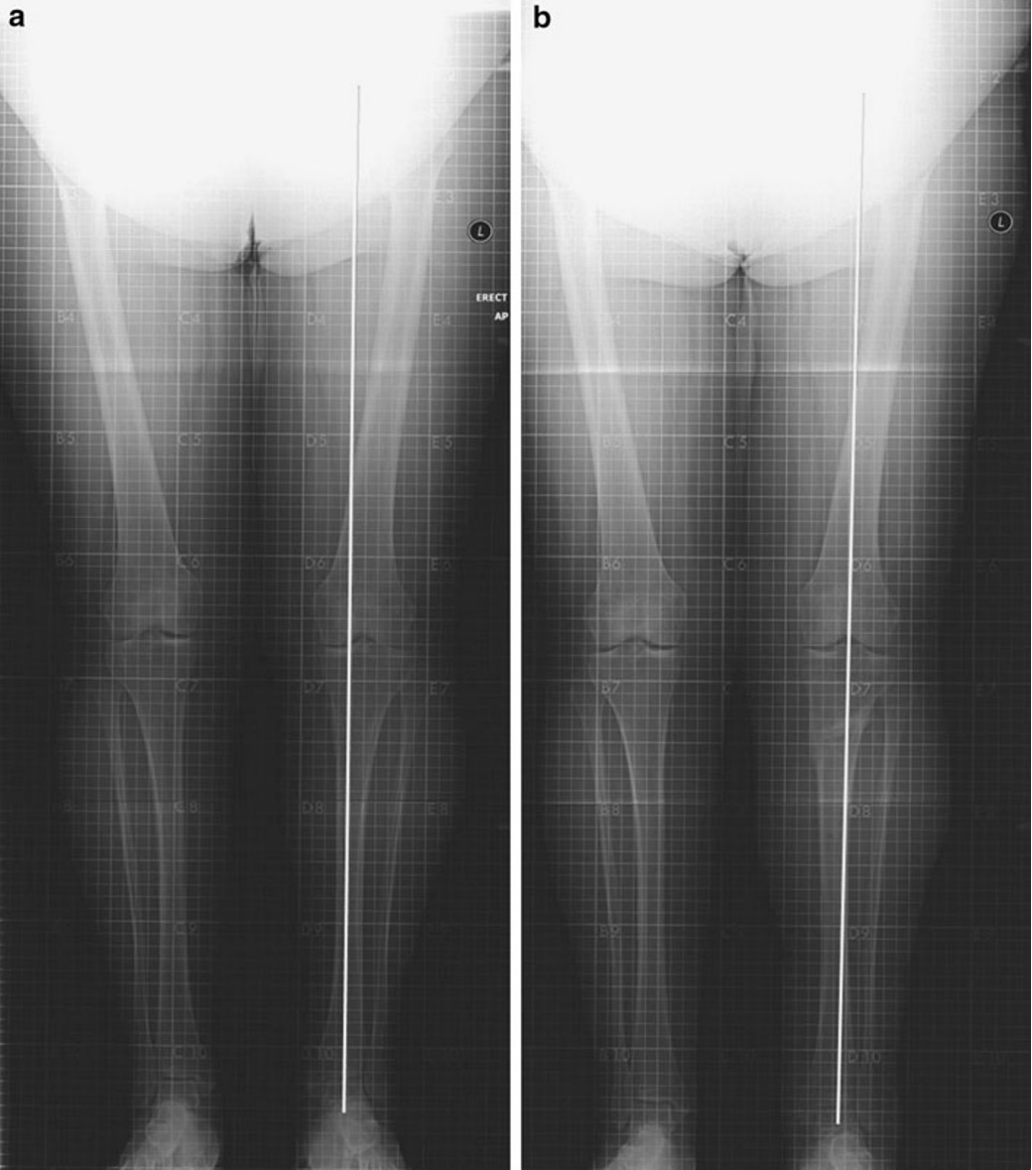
Long leg standing AP radiographs demonstrating mechanical axis. a preoperatively, b post-correction
